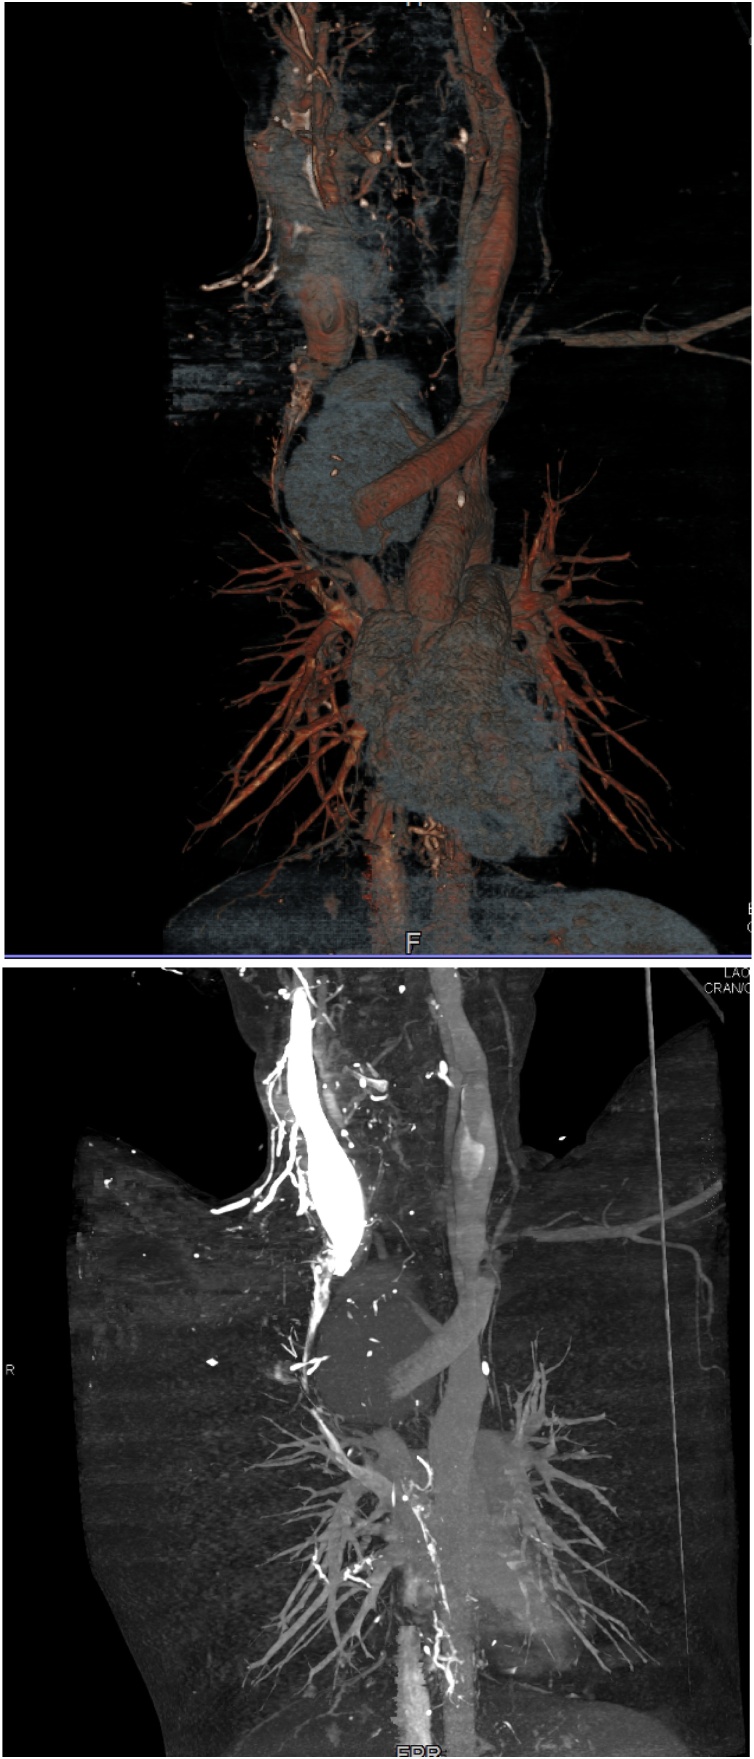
CT reconstruction images show the interruption of the innominate artery with the pseudoaneurysm. The right subclavian artery is not filling. The SVC is markedly compressed and thinned out.
